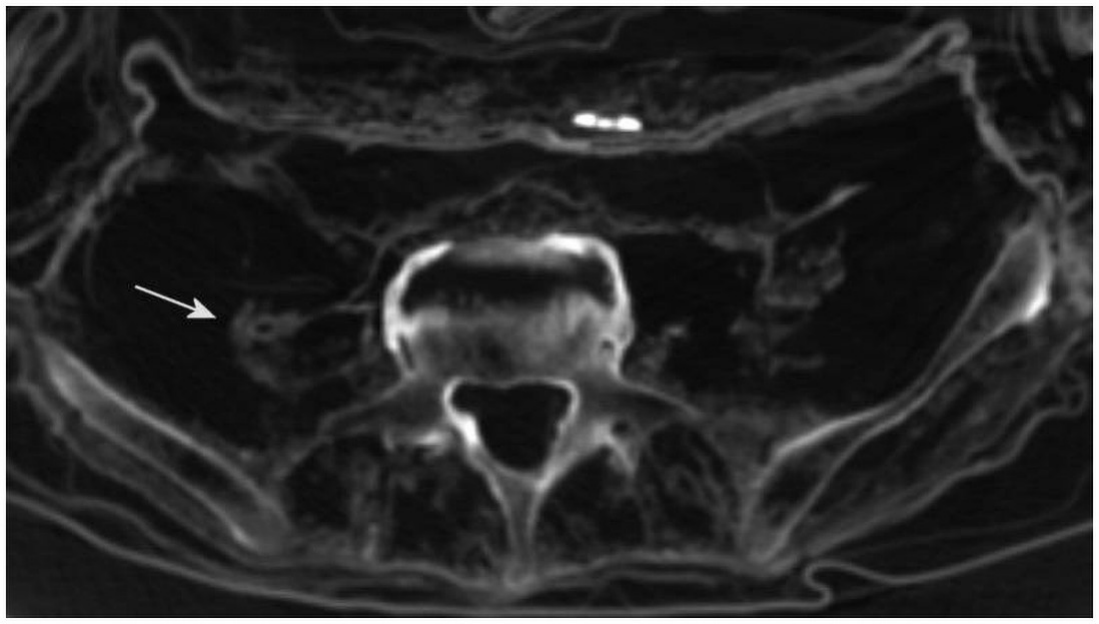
CT scan of a retroperitoneal mass on the right side (arrow).
